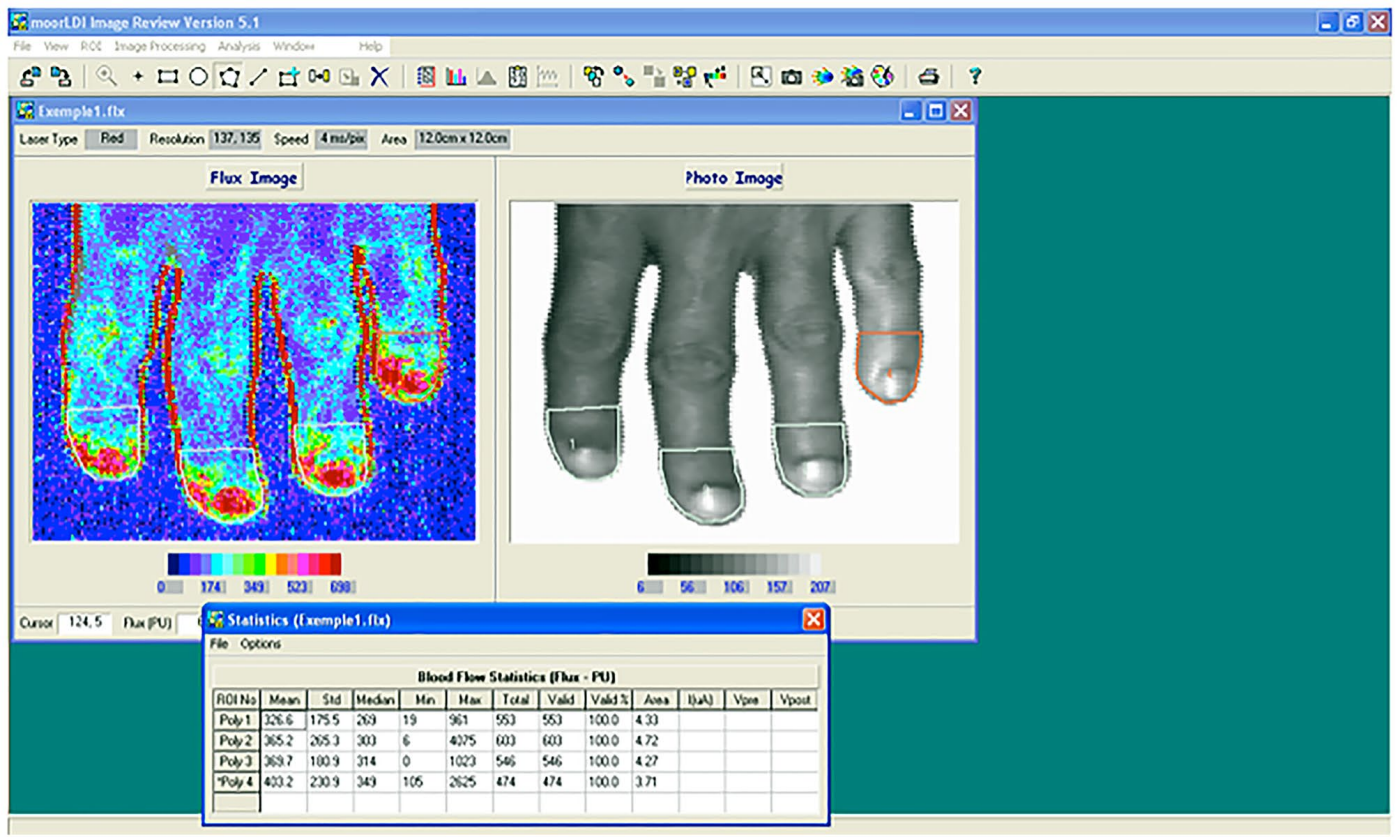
Laser Doppler imaging analysis of the distal phalanx (in this case of a patient presenting optic disc hemorrhage with an intraocular pressure of 18 mmHg).
